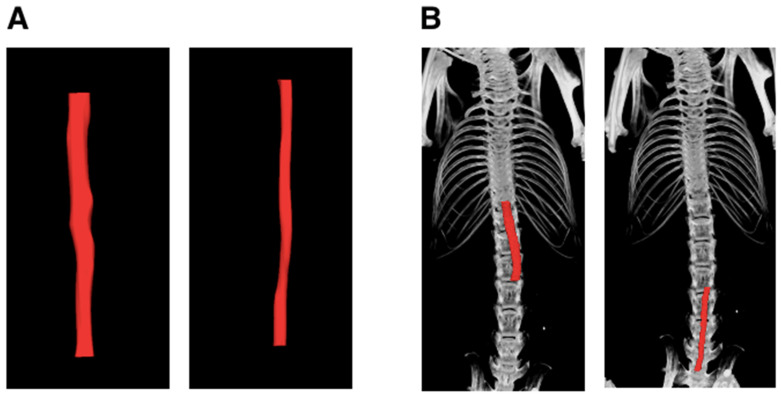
Images of suprarenal and infrarenal aortas reconstruction in (A) ultrasound (USS); suprarenal (left) and infrarenal (right) and (B) micro-computed tomography (μCT); suprarenal (left) and infrarenal (right).
